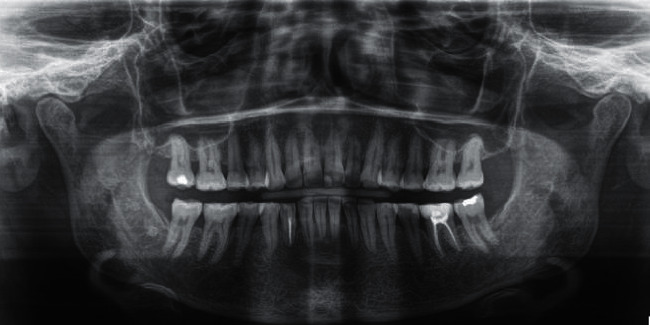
Final orthopantomography.
